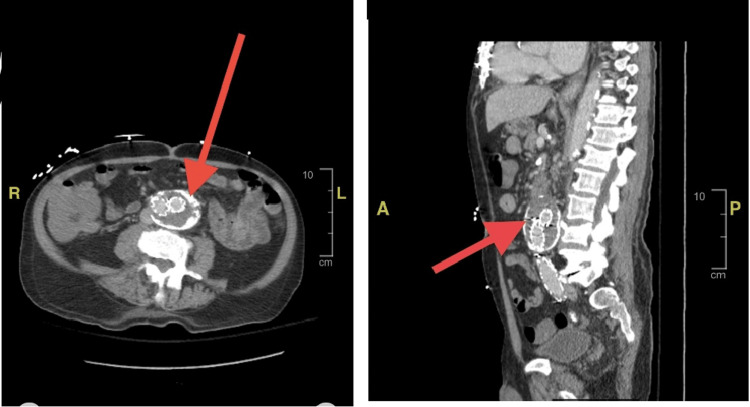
CT of the abdomen and pelvis with contrast on admission showing an infrarenal abdominal aortic aneurysm with the aortobiiliac stent graft now with inflammatory stranding in the proximal aspect of the graft and the aortic wall concerning for aortitis or an infected graft. The first image shows an axial view and the second image shows a coronal view. Both arrows point to the location with inflammatory changes.
